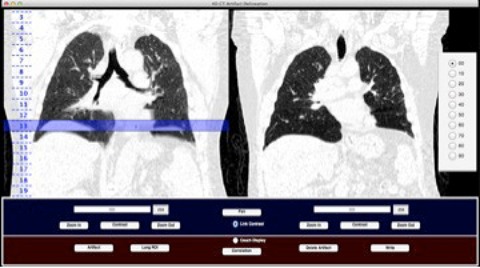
Artifact evaluation software showing: T0% of a 4D CT scan (left) with a highlighted identified artifact at couch position 13 indicating a saved artifact location; and corresponding deep‐inspiration breath‐hold scan (right) used as an anatomic reference for artifact identification in the 4D CT (left).
